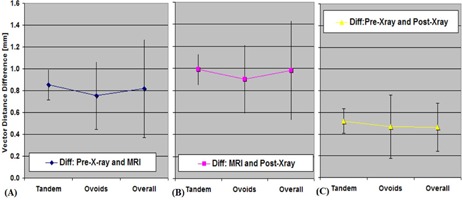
The values of Δd for 19 T&O plans (a) between pre‐X‐ray and MRI; (b) between MRI and post‐X‐ray; (c) between pre‐X‐ray and post‐X‐ray The overall reconstruction uncertainties of T&O on MRI are on average less than 1.1 mm regardless of control datasets (i.e., pre‐ or post‐X‐ray). In addition, panel (c) demonstrates the overall reconstruction uncertainties of T&O between two control X‐ray datasets are on average less than 0.6 mm.
